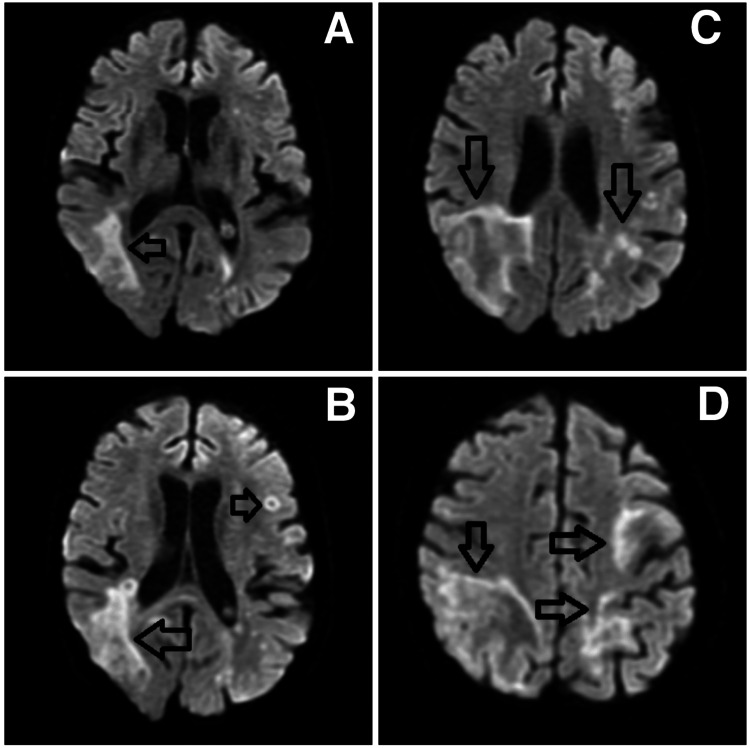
(A-D) Magnetic resonance imaging of the brain showing right parietotemporal white matter T2 hyperintensity (DWI sequence) and suggesting an inflammatory/demyelinating process. The relative lack of mass effect and DWI leading edge suggest progressive multifocal leukoencephalopathy. There are similar changes on the left in the frontal and parietal lobes. There is a mass effect associated with the white matter change, but it is modest. There is a leading edge of diffusion restriction associated with the hemispheric white matter change and some definite rings of restriction. DWI, diffusion-weighted imaging
